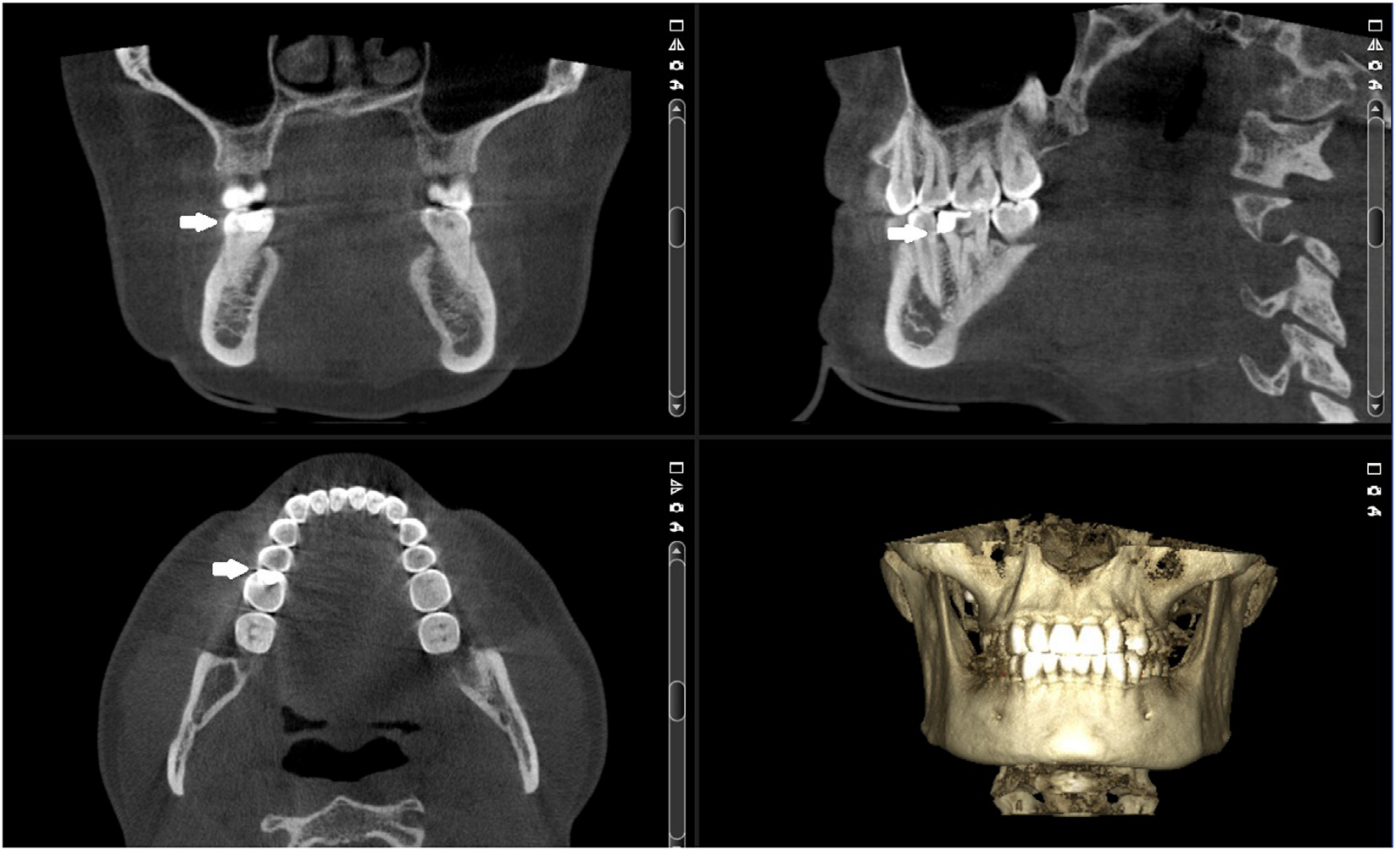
Coronal, sagittal, and axial CBCT images of an approximal restoration exhibiting a distinct step or ledge of filling material extending beyond the normal smooth profile of a mandibular right first molar.
